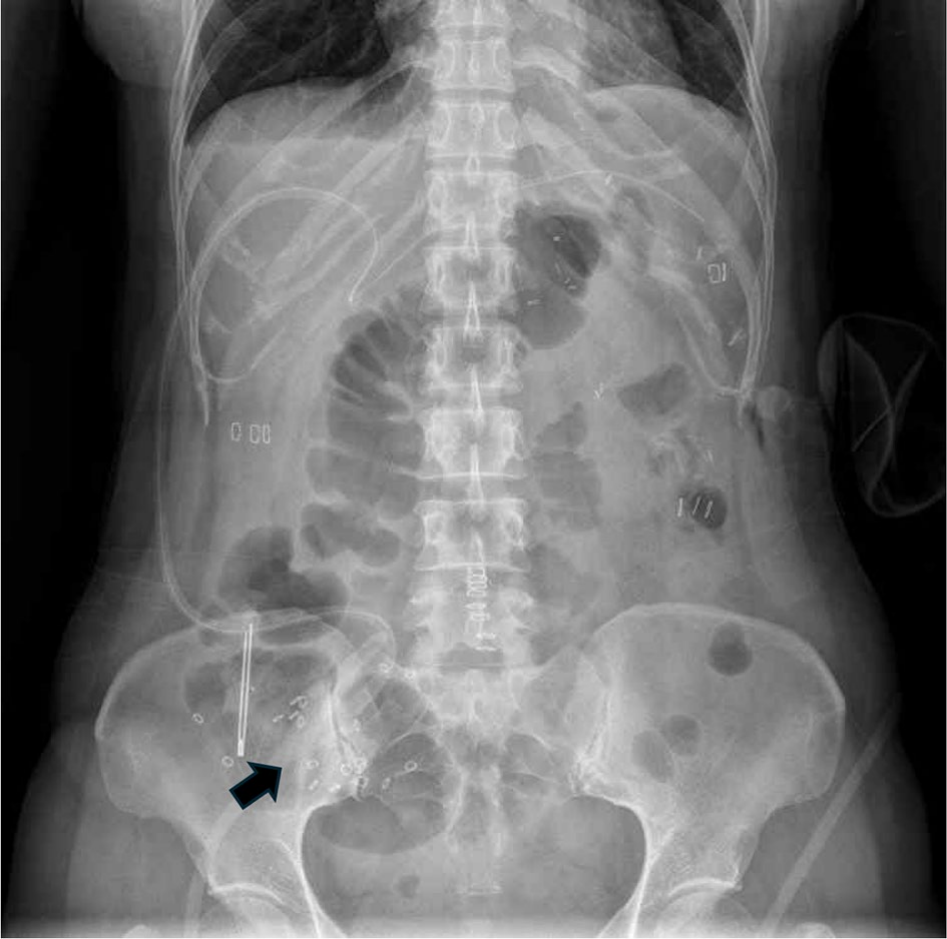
Representative radiographic image of radiopaque markers observed in the colon. (Black arrow).
